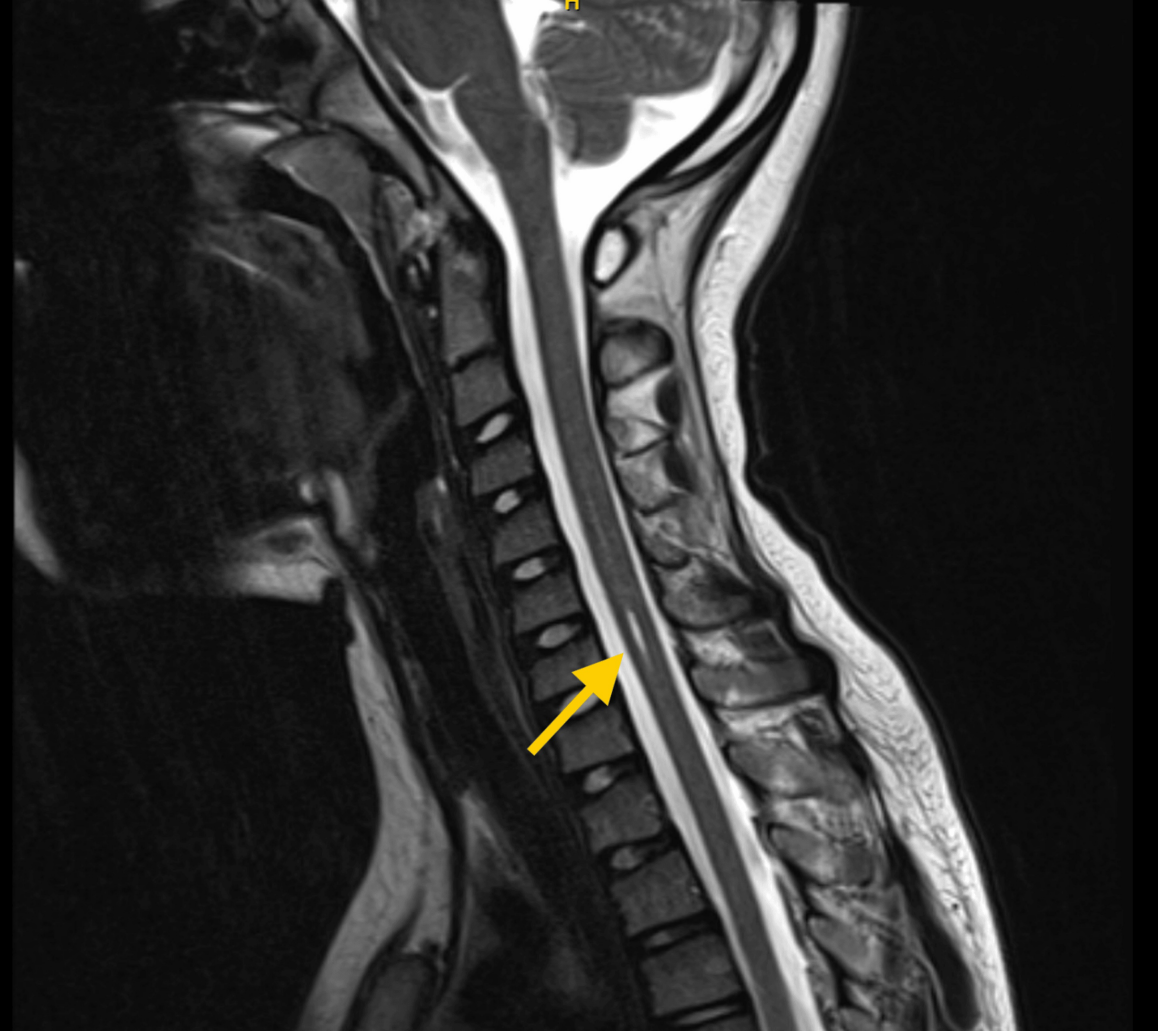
MRI T2-weighted image of cervical syringomyelia (arrow) MRI: magnetic resonance imaging
